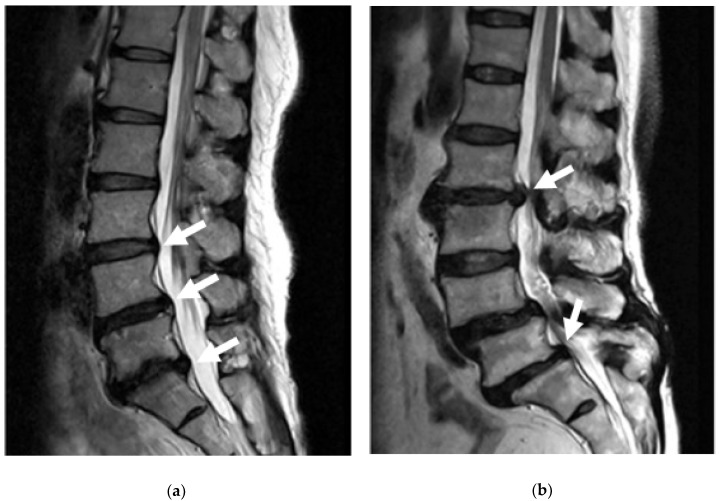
MRI image showing a slight bulge of the annulus into the spinal canal without severe impingement (a). MRI image showing a full lumbar disc herniation with substantial spinal stenosis and nerve-root compression (b) [97].
